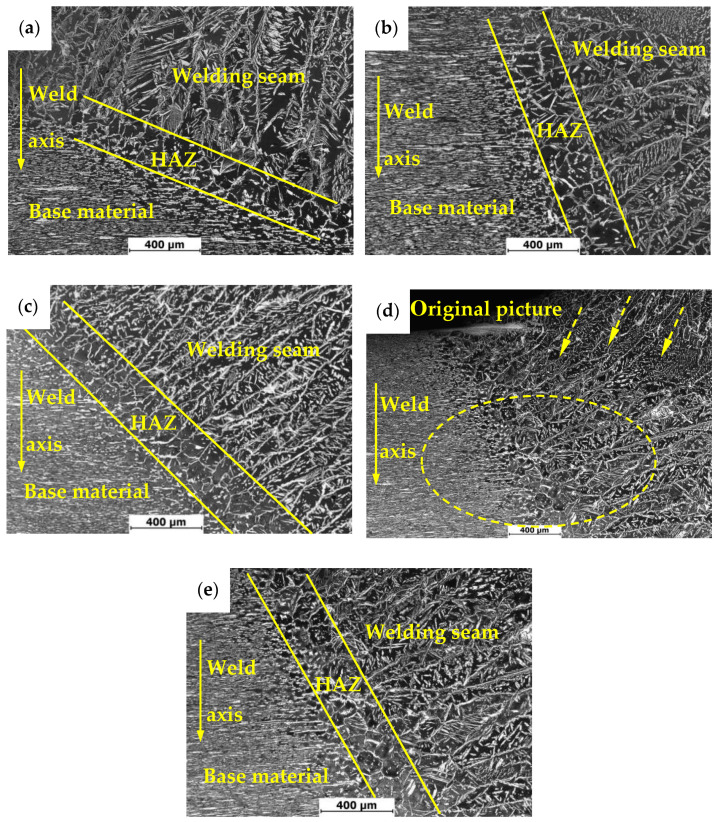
Metallographic structure of DSS 2205 heat-affected zone with different treatment methods: (a) untreated; (b) pneumatic chipping hammer; (c) ultrasonic peening treatment; (d,e) heat treatment.
